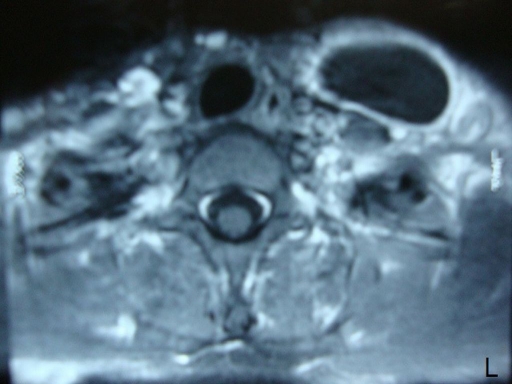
Contrast-enhanced axial T1-weighted MRI showing a well-defined hypointense cystic mass with a periferic enhancement.
